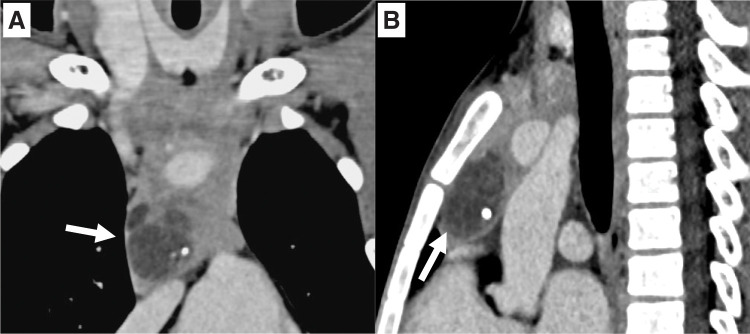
Contrast-enhanced chest CT. (A) Coronal view showing an anterior mediastinal mass with cystic components (white arrow). (B) Sagittal view demonstrating the cystic portion of the tumor (white arrow).
